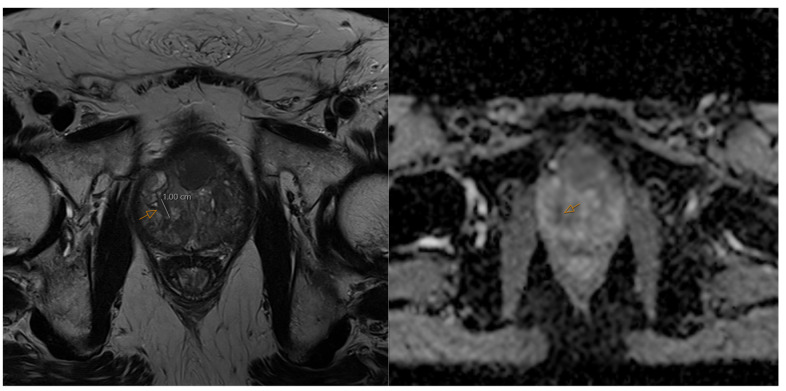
In this mpMRI prostate, the arrow shows on axial T2 sequence (left) and an axial DWI sequence (right) a 1.0 × 0.8 cm right posterior apex transitional zone Gleason 6 lesion in a 69-year-old prostate cancer patient with diabetes.
